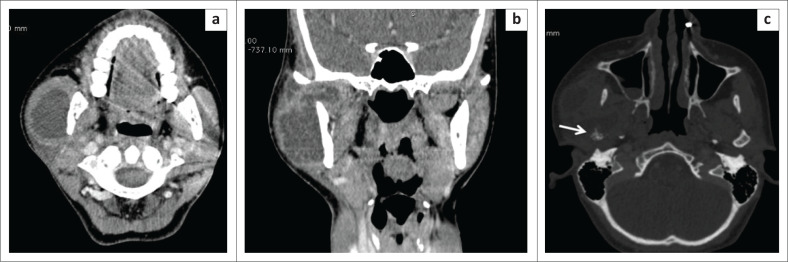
A 23-year-old female with swelling in the right parotid region. Post-contrast axial (a) and coronal (b) computed tomography images demonstrate a hypodense collection in the right masseter extending into the temporo-mandibular joint. Axial computed tomography bone window image (c) shows the involvement of the right mandibular condyle with partial lysis of the bone and cortical erosion (white arrow).
